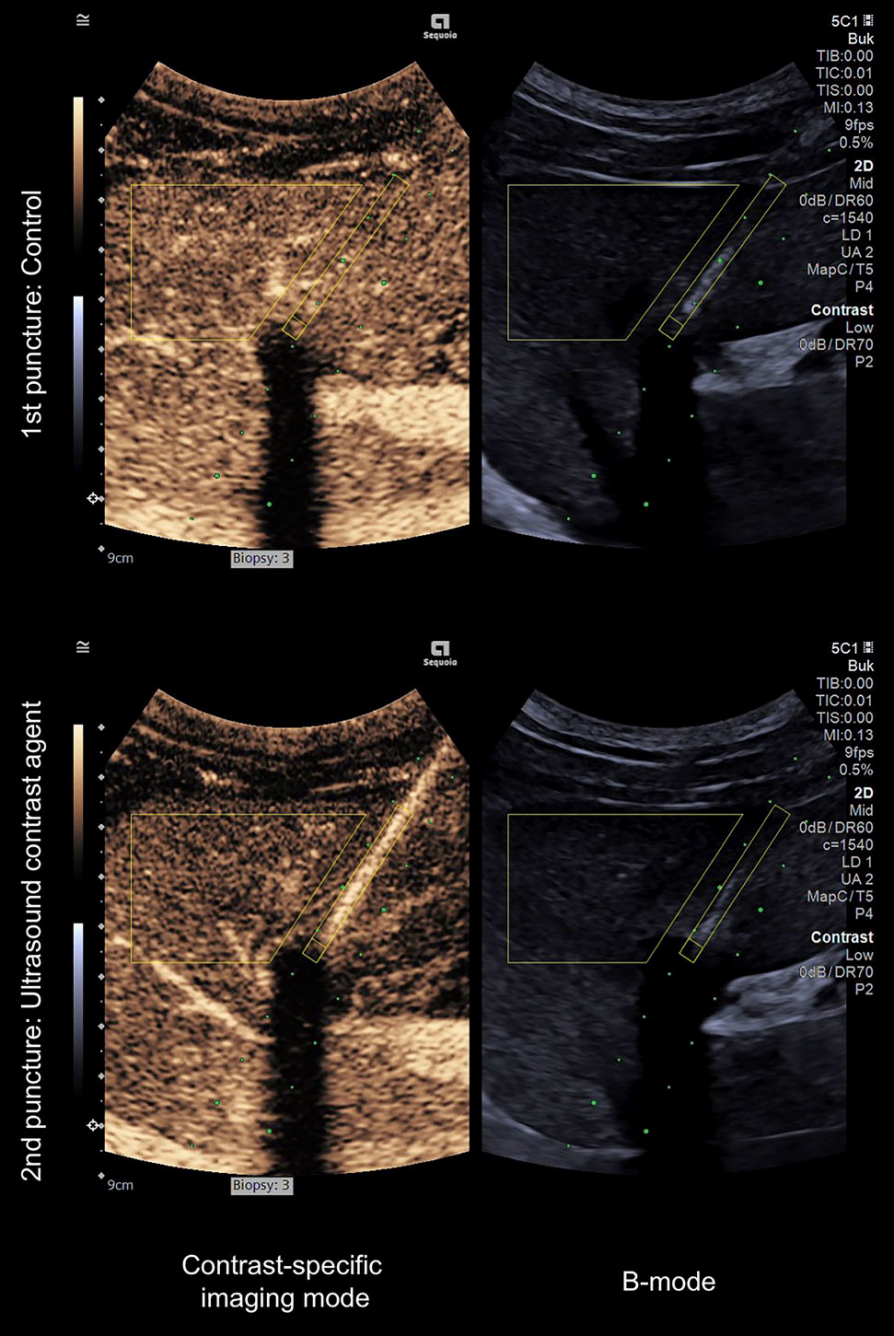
Example of a pair of punctures (third pair of the fifth set) with needles in their end positions adjacent to the thoracic drain. Yellow boxes represent the two regions of interest and the background, as described in detail in Fig. 2. The pair was chosen to represent a contrast-to-noise ratio near the median in contrast-specific imaging mode for the needle in its entirety of the ultrasound contrast agent primed needles
